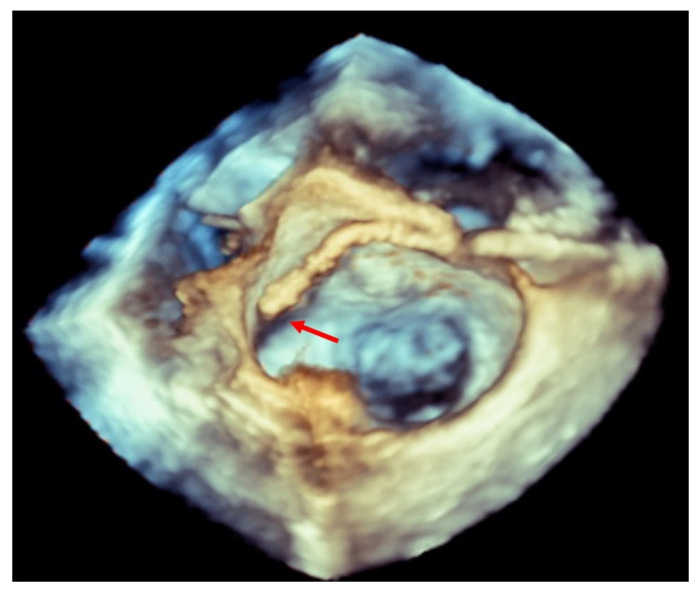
Three-dimensional transesophageal echocardiogram showing a clip inside the left atrium touching the lateral wall (red arrow).
